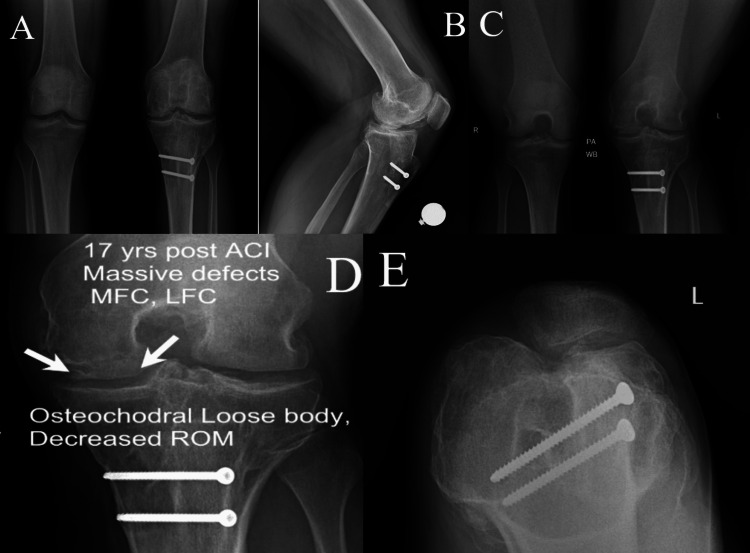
Anterior-posterior (A), lateral (B), posterior-anterior (C +D), and sunrise (E) radiographs of the patient’s knees upon initial examination; panel D is increased magnification of panel C with text superimposed to highlight the presence of an osteochondral loose body
